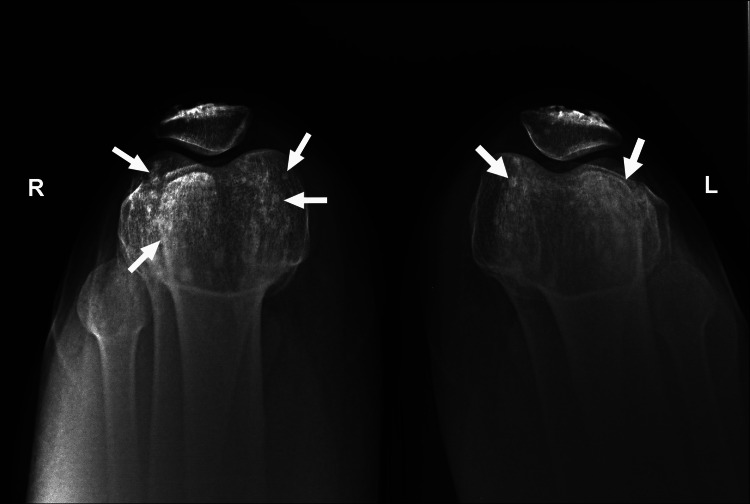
X-ray skyline view of the bilateral knee joint White arrows mark the bony lesions. A characteristic finding noted here is that there is no break in the cortex of the involved bones, including the patella. R: right lower limb; L: left lower limb
